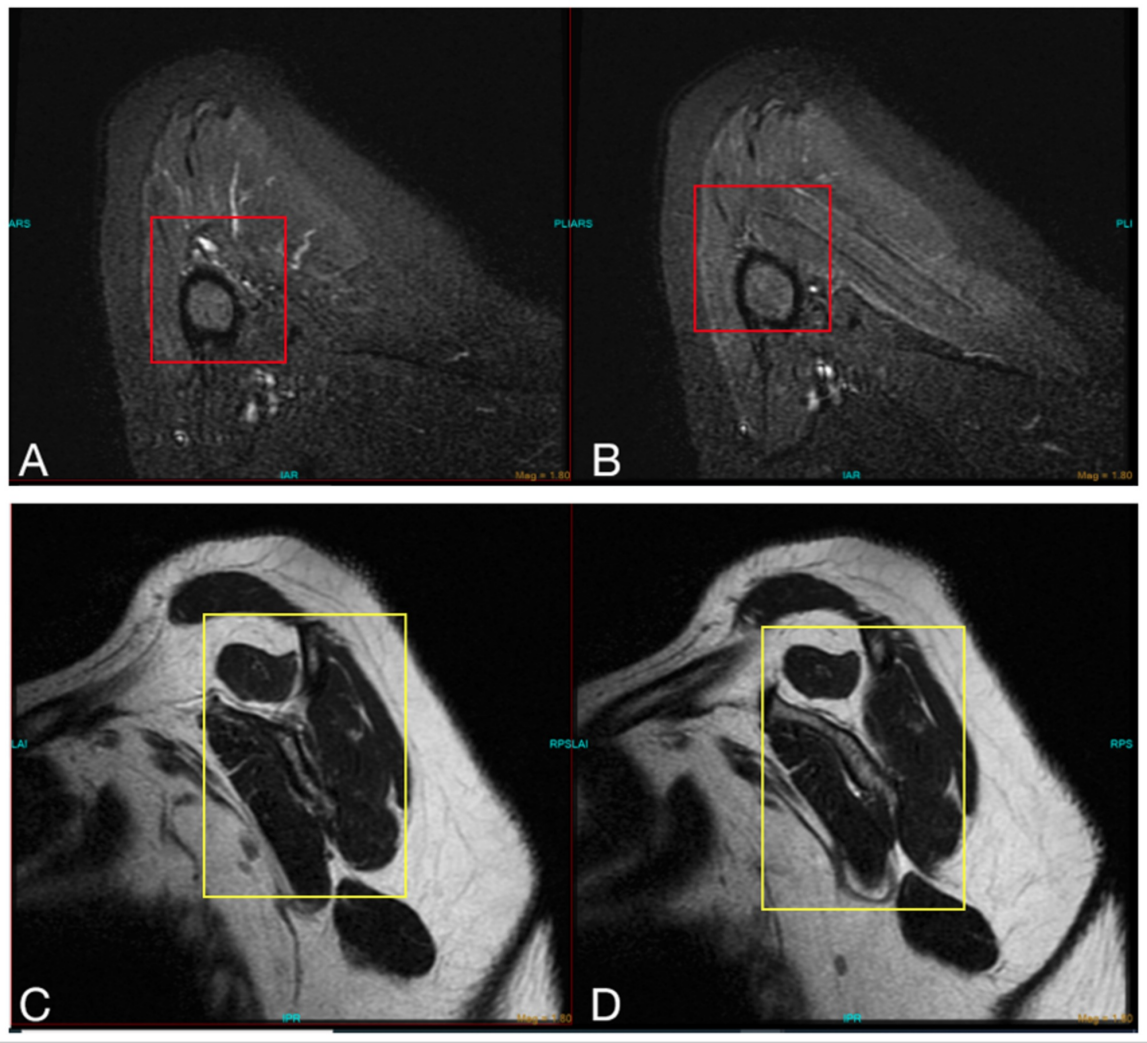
MRI of the right shoulder with multiple views demonstrating (A and B) mild rotator cuff tendinopathy with a partial thickness rotator cuff tear (red box) and (C and D) inflammation of the biceps muscle (yellow box).
